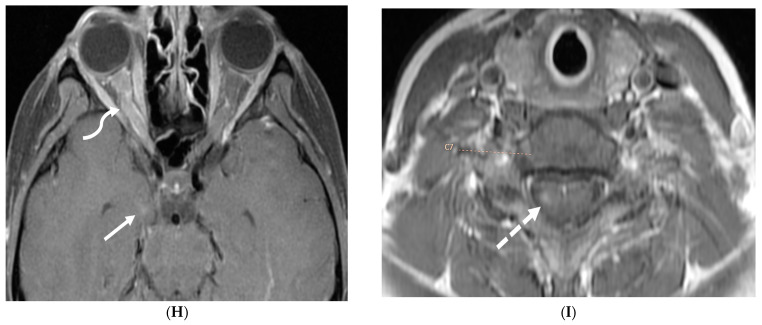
Sagittal T2 (A,B), axial FLAIR (C), axial T2 cervical spine (D) at the level of C7 vertebral body and Axial T2 orbits (E): 12-year-old girl presented with right focal motor seizure and left temporal lobe slowing on electroencephalogram (EEG). Right eye vision loss and irritability. Ill-defined areas of signal abnormalities are identified within the RIGHT mesial temporal lobe and bilateral medulla (white arrows). FLAIR hyperintensity is identified on the left central sulcus (black arrow). Small focus of signal abnormality is seen on the right side of the cord at the 7th cervical vertebra (C7) (dashed arrow). There is also bilateral papilledema (arrowheads). Post contrast axial T1 (F,G), axial T1 orbits (H) and axial T1 cervical spine at C7 (I): Asymmetric LME (black arrows) predominantly involving the left cerebral hemisphere, with minimal right parietal involvement is seen. Ill-defined enhancement in the right mesial temporal lobe, and right greater than left medulla (white arrows) corresponds to the signal abnormality. There is right greater than left, optic nerve enhancement (curved arrow). Single small enhancing lesion in the spinal cord on the right at the level of C7 corresponds to the signal abnormality (dashed arrow). Features favor a demyelinating process. MOG antibodies were positive at 1:20 in keeping with Myelin oligodendrocyte glycoprotein (MOG) antibody disease (MOGAD).
